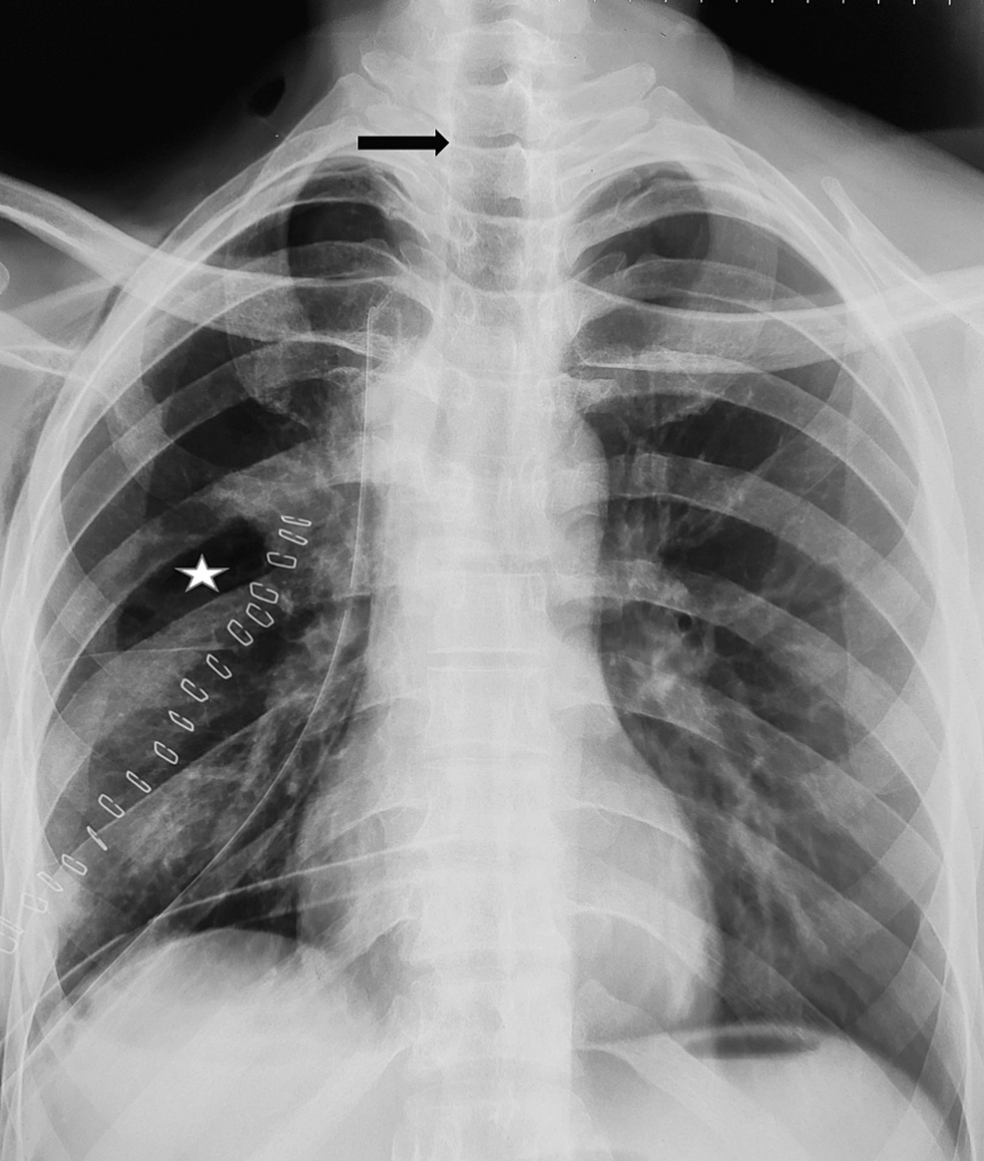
Postoperative chest X-ray showing an expanded right lung (star) with midline trachea (arrow), consistent with a successful bronchial anastomosis
